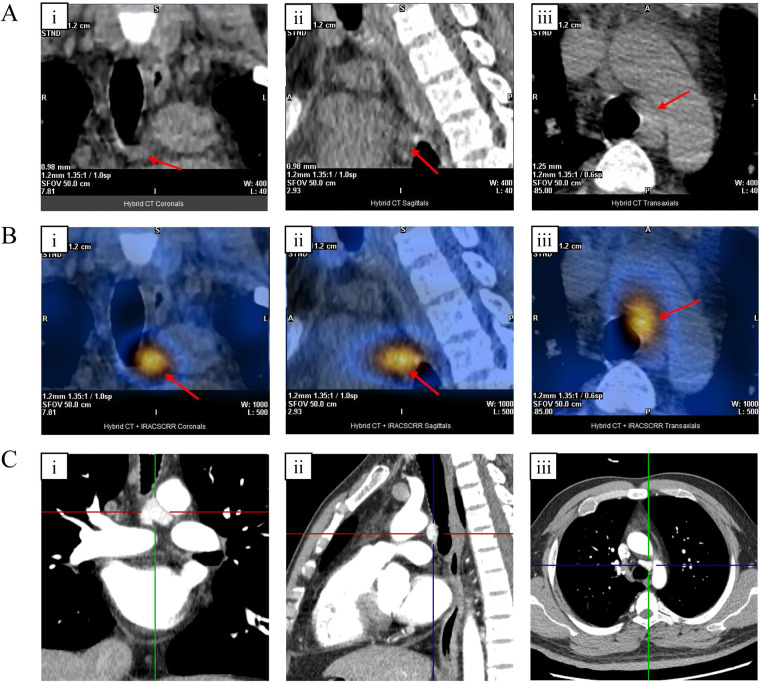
(A) Parathyroid SPECT/CT (CT component): coronal (i), sagittal (ii), and axial (iii) views demonstrating a solid nodule with well-defined borders and slightly heterogeneous density in the superior mediastinum, located anterior to the left side of the trachea and inferior to the aortic arch. (B) 99mTc-MIBI SPECT/CT (Fusion): Corresponding functional images (i–iii) revealing focal increased radiotracer uptake in the nodule, indicating hyperfunctioning parathyroid tissue. (C) Coronal (i), sagittal (ii), and axial (iii) multiplanar reconstruction views identifying a triangular soft-tissue mass (approx. 21 mm × 15 mm × 20 mm) in the same region.
